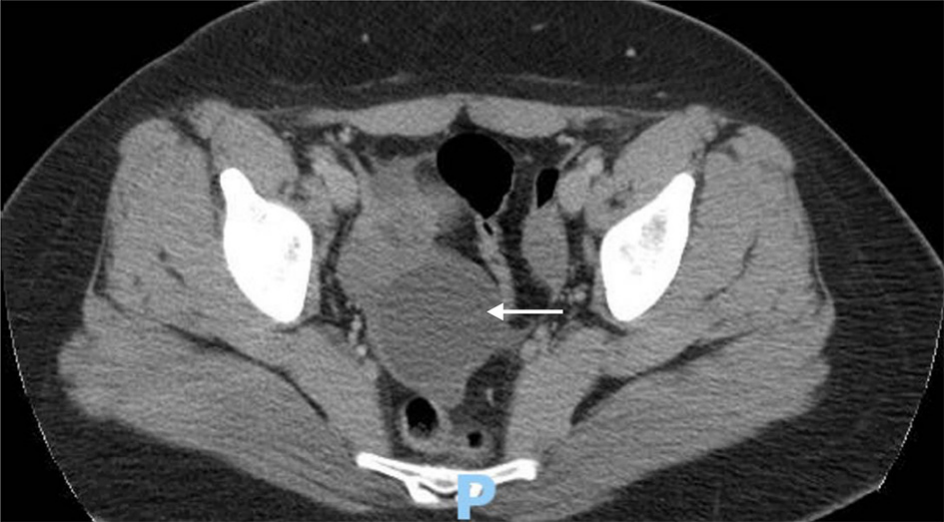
CT abdomen/pelvis with IV contrast demonstrates right adnexal cyst. Ovary in this image unable to be visualized.
